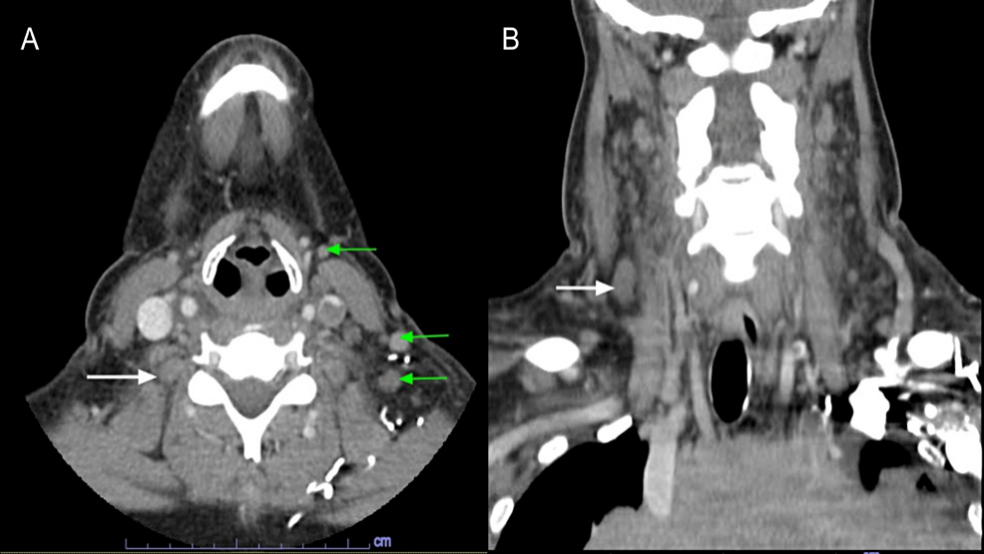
CT head and neck with contrast (A: axial view; B: coronal view) showed bilateral enlarged cervical lymph nodes (green arrows), with the largest within the right internal jugular chain (white arrows).
